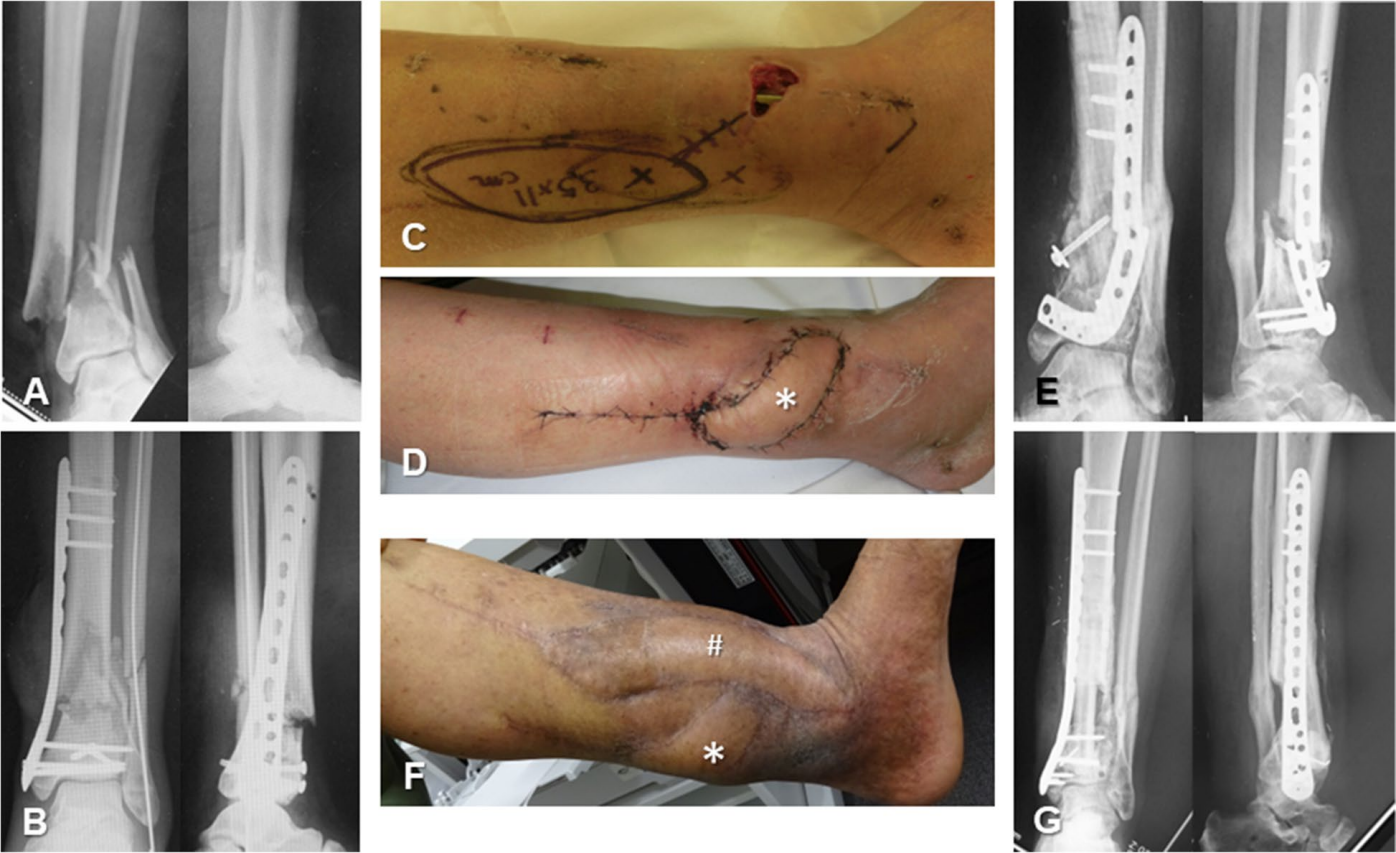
A A-65-year-old man with an open distal tibial fracture. B, C After osteosynthesis with an anterolateral plate, a 3 × 3 cm soft tissue defect with plate exposure remained on the anterior surface of the tibia. D Three weeks after osteosynthesis, soft tissue reconstruction was performed using a tibialis posterior perforator-based propeller flap (asterisk). The flap survived intact without complications such as congestion, but osteomyelitis occurred at 5 months after insertion of the propeller flap. Debridement and re-osteosynthesis with an autogenous iliac bone graft were performed. E X-rays 1 year after re-osteosynthesis. Implant breakage caused by infectious pseudoarthrosis was confirmed. F Bone reconstruction was performed using a contralateral free vascularized fibula bone graft (hashmark). G X-rays 2 years after insertion of the vascularized bone graft showed complete bone union
